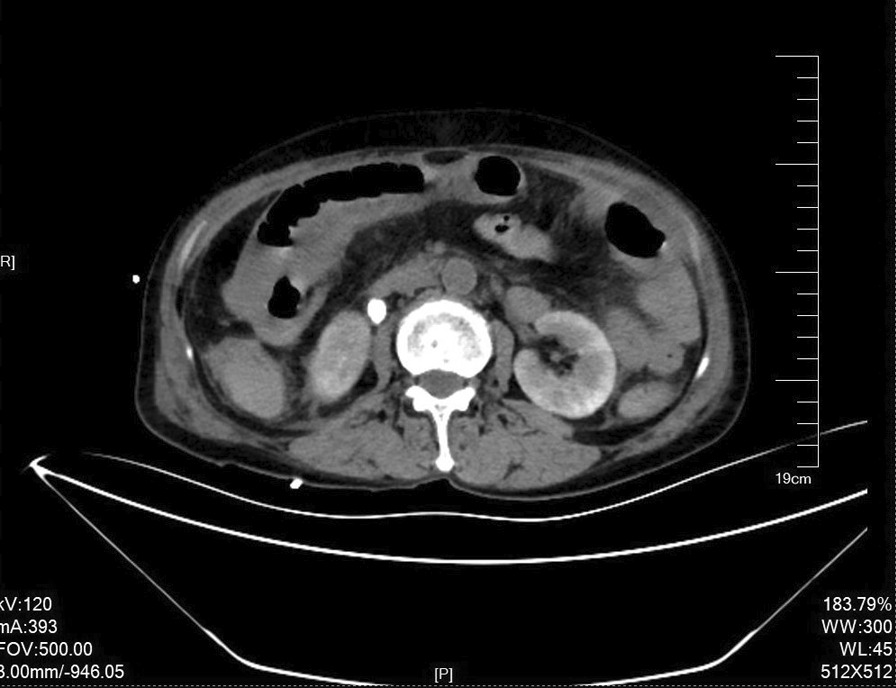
Abdominal CT-scan: intestinal dilatation, intestinal wall edema, and mesenteric exudation. Free fluid in the abdominal and pelvic cavity. Right lateral upper ureteric calculi measuring approximately 9 mm * 10 mm with right upper ureteral dilatation and hydronephrosis
